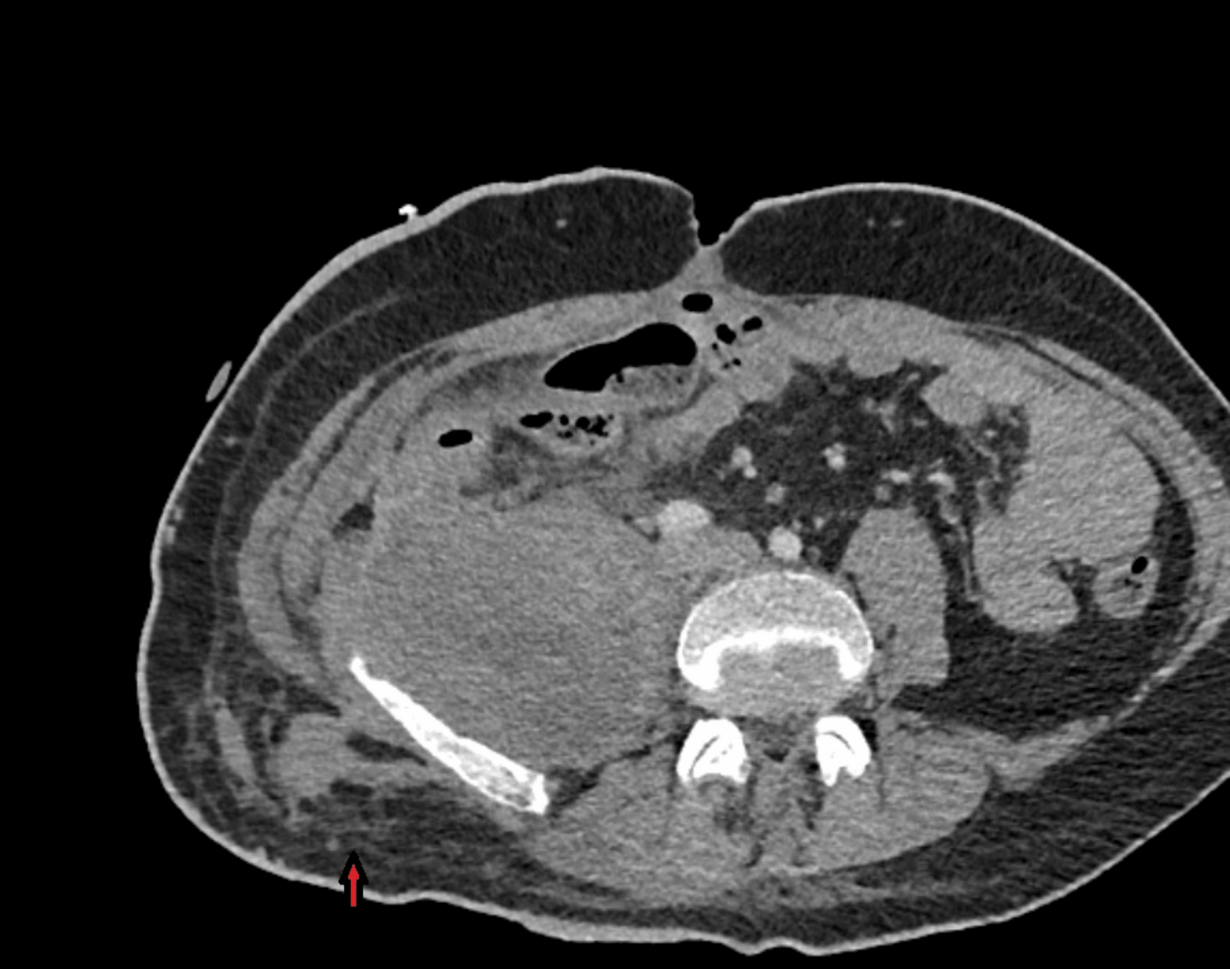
Postoperative CT imaging after 1 year showing the healed area (red arrow).
